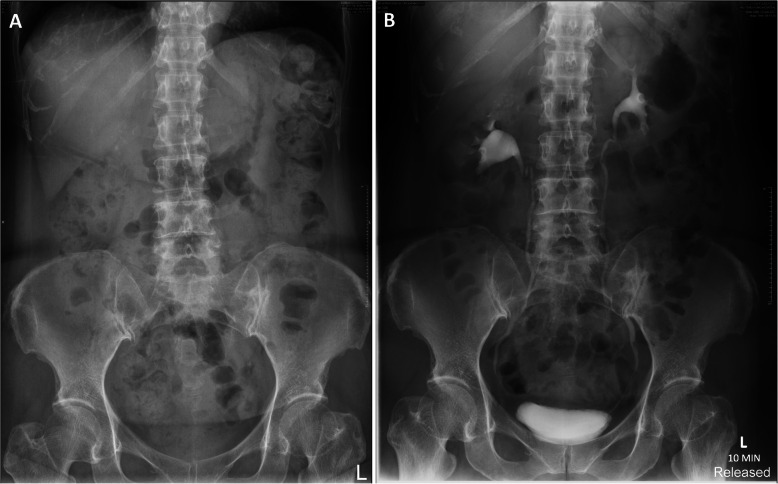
Interval plain radiograph performed the following day (a). Follow-up intravenous urography performed a month later (b). Both show no further contrast extravasation or persistent contrast pooling
